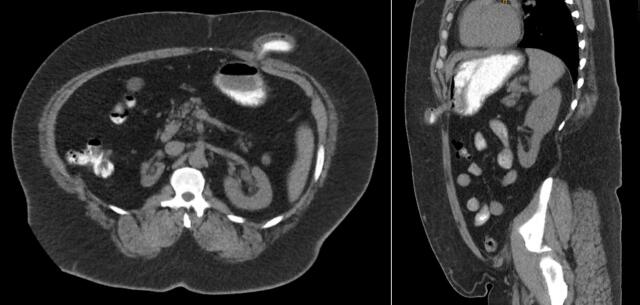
CT abdomen/pelvis scan with oral gastrogaffin contrast demonstrating a patent GCF.
